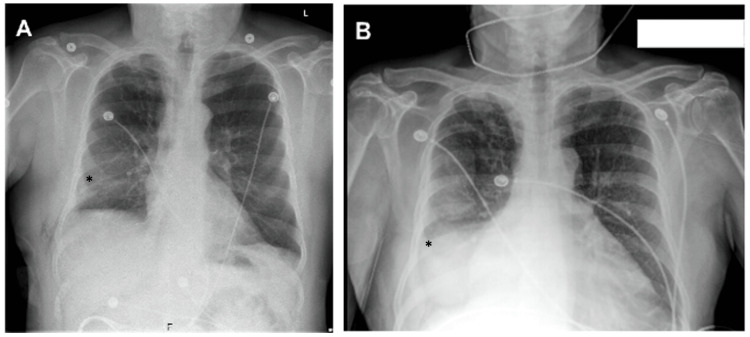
(A) Chest X-ray obtained postoperatively following segmentectomy and chest tube removal revealing normal right-sided subsegmental atelectasis (*). (B) Chest X-ray obtained on return to the emergency department revealing a new right pleural-parenchymal reaction (*) concerning for infection and/or pleural effusion.
